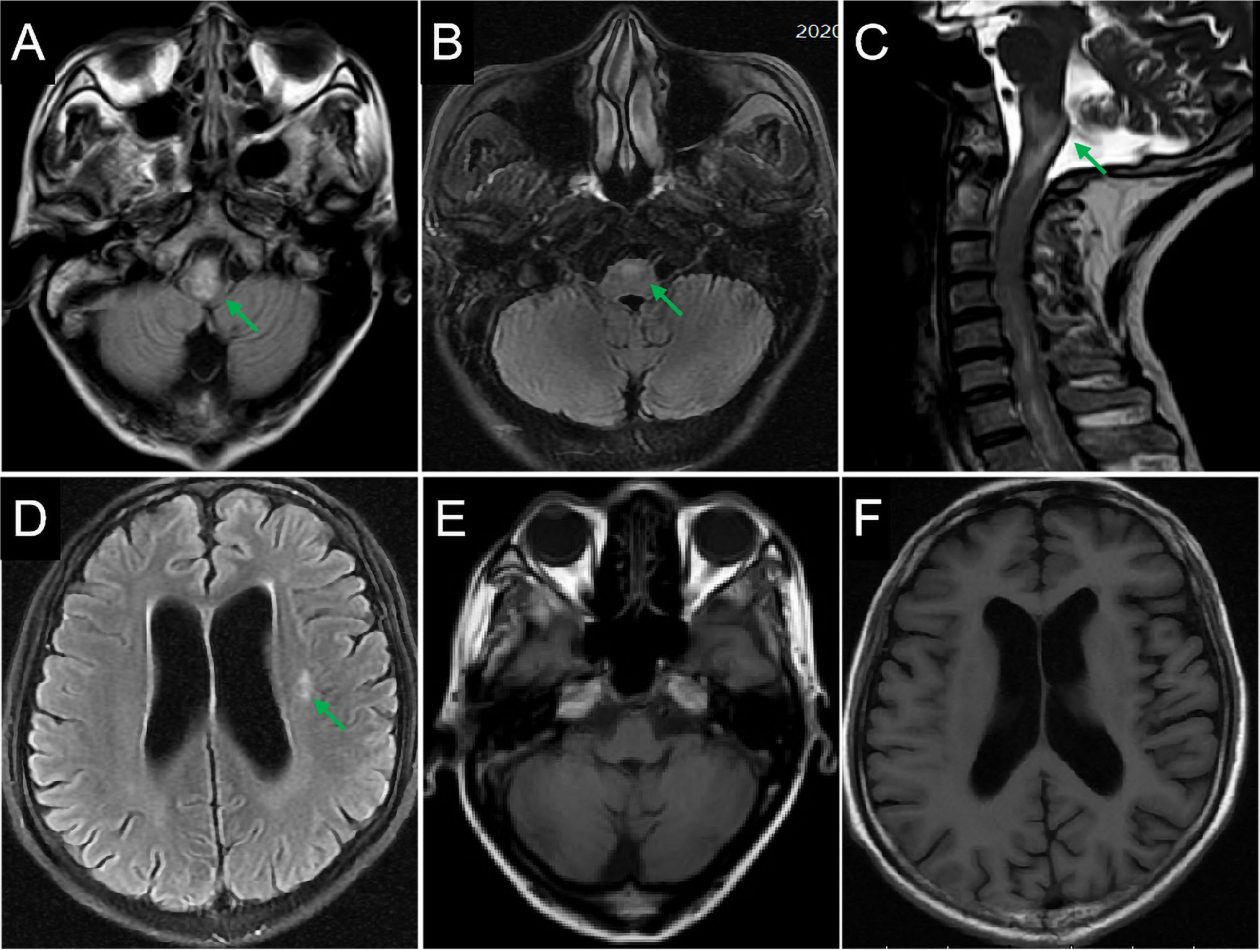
Brain magnetic resonance imaging. T2-weighted imaging (WI) showing hyperintense lesions in the central medulla oblongata (A, B, C, arrows), inferior-middle part of pons (C, arrow), and left ventriculus lateralis (D, arrow). There was hypointensity (E, F) in the corresponding part on T1-WI imaging
